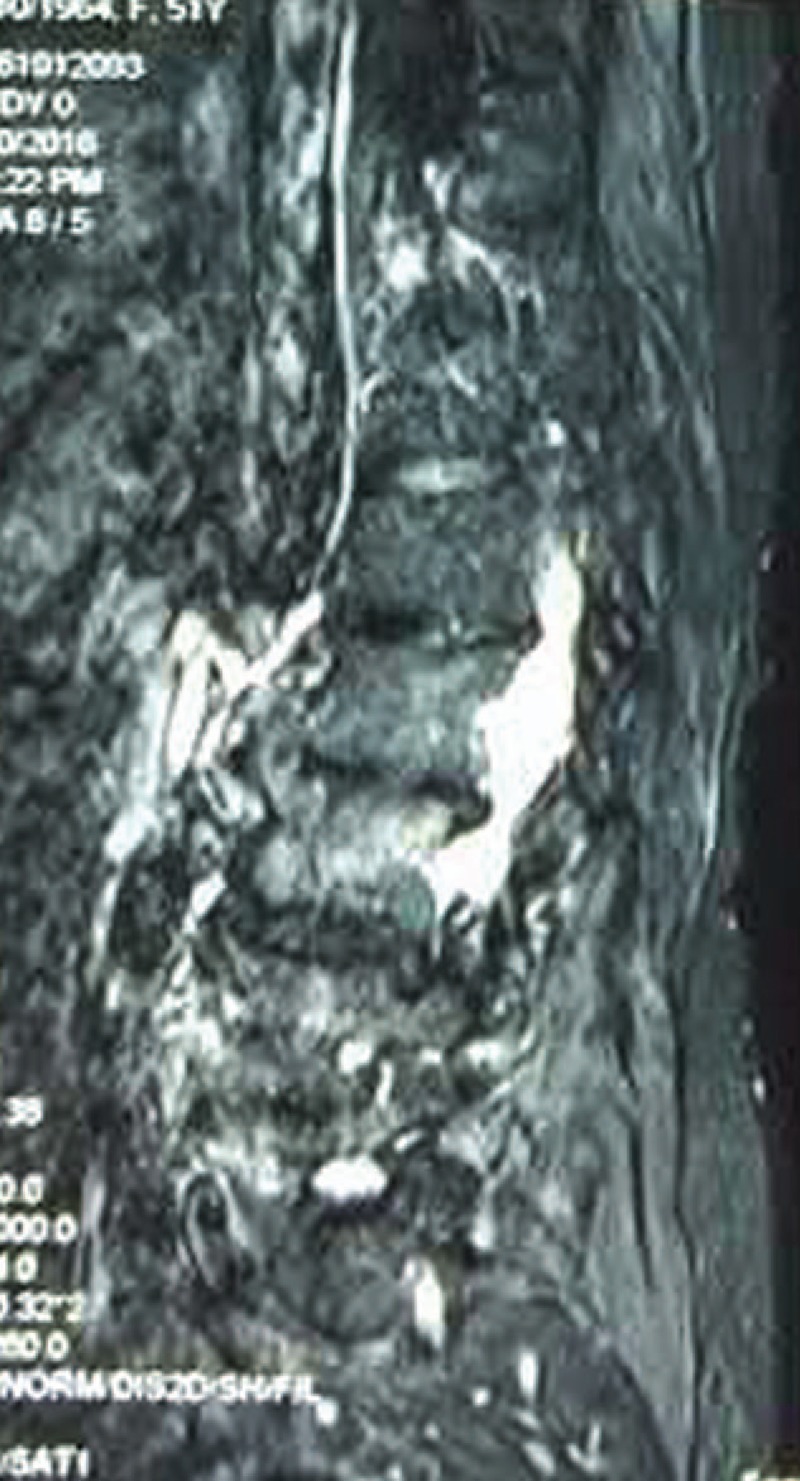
Positive and lateral full spine x-ray before surgery. PI = 60°, PT = 30°, SS = 30°, LL = −20°, T5-T12 = −10°, SVA = 75 mm. PI = pelvic incidence, PT = pelvic tilt, PT = pelvic tilt, SS = sacral slope, SVA = sagittal vertical axis from C7 plumb line.
